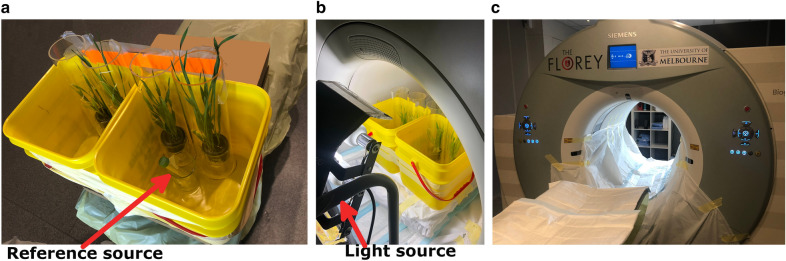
Experimental setup. a Four plant setups in two larger containers with the reference source, b Experimental setup in the scanner with the light source on, c Siemens Biograph128 mCT PET/CT scanner
